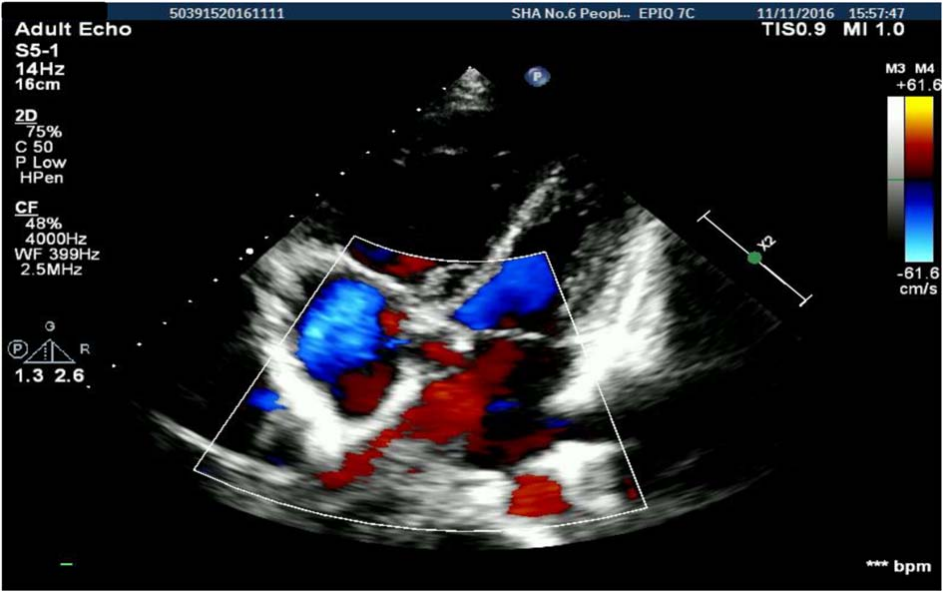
Transthoracic echocardiography suggested secondary atrial septal defect.
